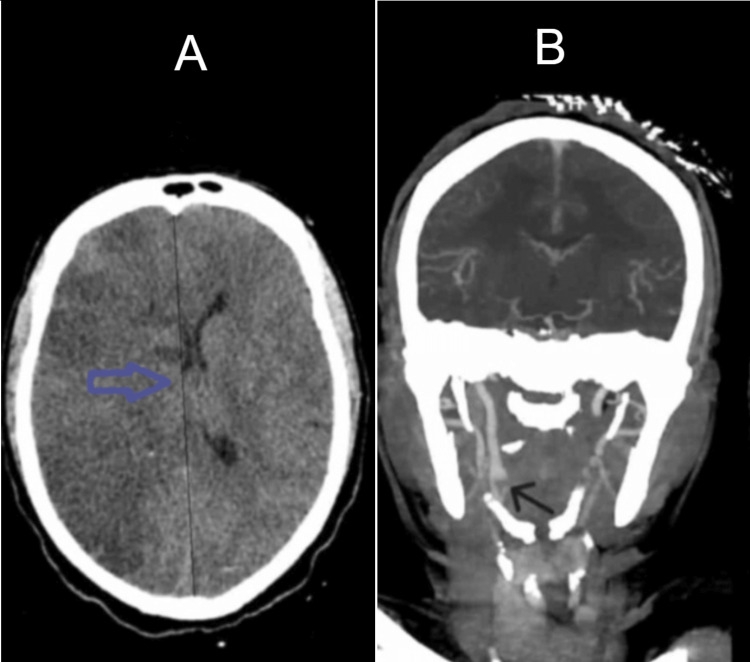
(A) Non-contrast CT head showing malignant infarction of the right MCA territory with ~3 mm midline shift (blue arrow). (B) CT angiography demonstrating thrombus in the right internal carotid artery (black arrow). MCA: middle cerebral artery
